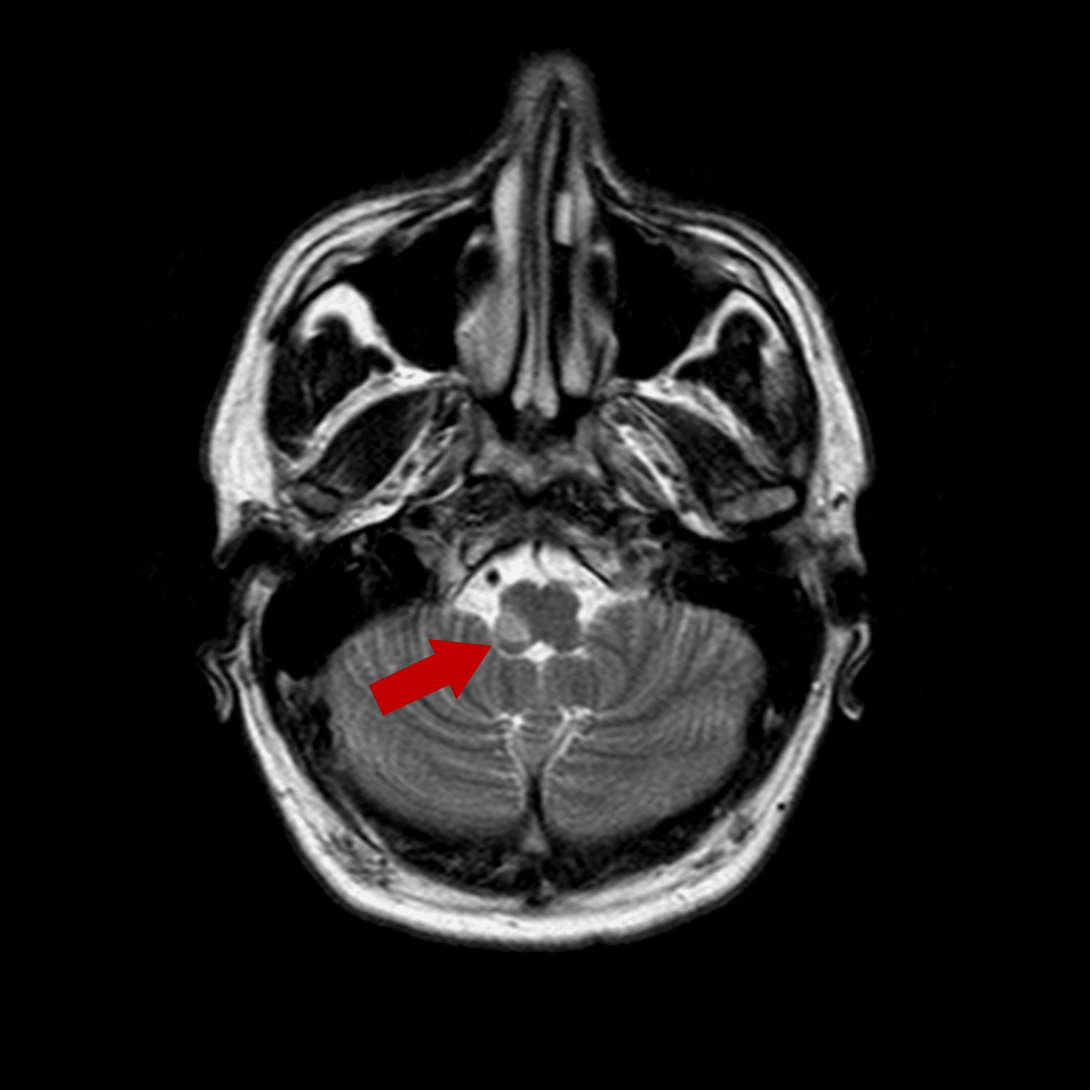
Brain MRI Axial diffusion-weighted MRI reveals a hyperintense lesion involving the right lateral medulla (red arrow), corresponding to restricted water diffusion consistent with acute infarction in the territory of the right vertebral and posterior inferior cerebellar arteries. This radiological pattern is characteristic of lateral medullary (Wallenberg) syndrome and explains the patient’s dysphagia, dysphonia, and contralateral sensory deficits.
